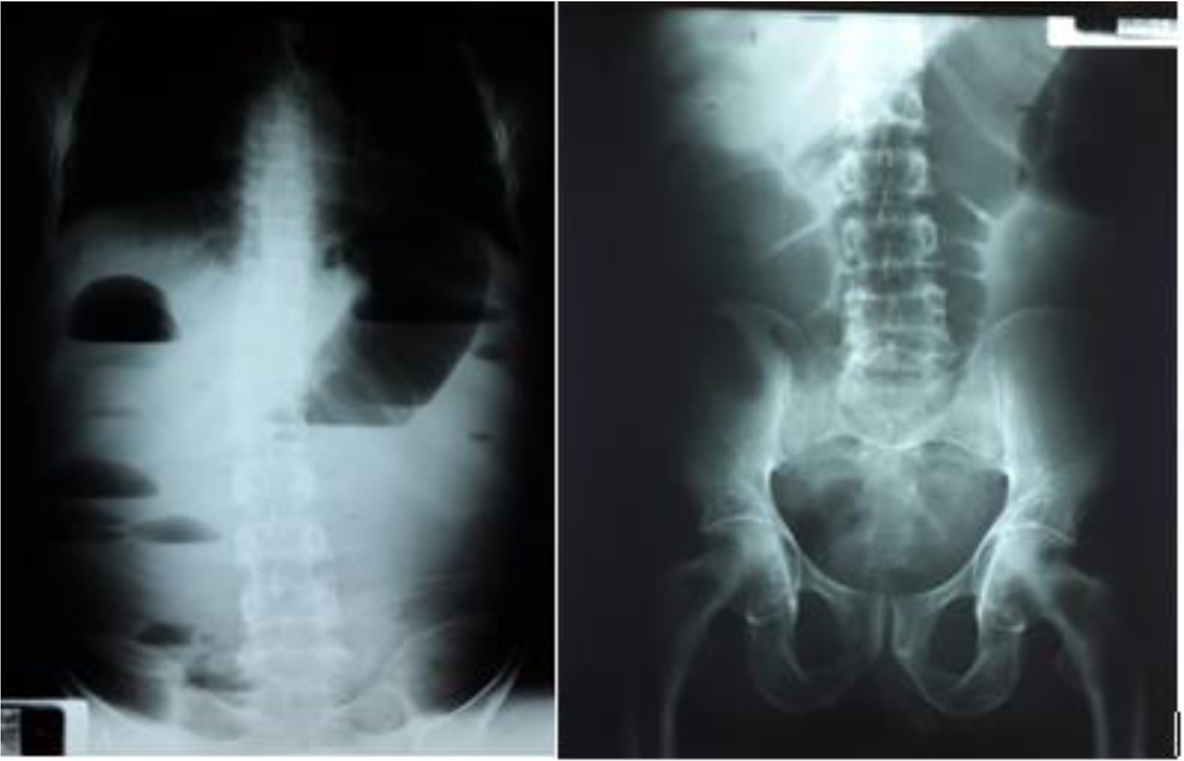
Plain abdominal X-rays showing multiple air fluid levels with dilated large bowel
